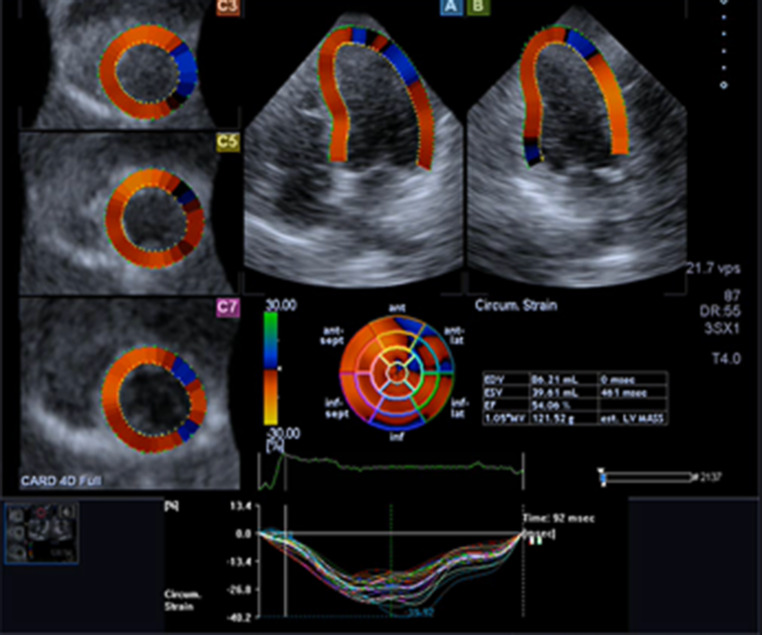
Measurements of the left ventricular (*LV*) volumes and function using three-dimensional speckle tracking echocardiography. Example of multiple long- and short-axis views extracted from a three-dimensional echocardiography data set at end-systole in a patient with normal systolic LV function. Visual information on circumferential (*Circum.*) strain is shown in colour overlay superimposed on grey-scale images (*EDV* end-diastolic volume, *ESV* end-systolic volume, *EF* ejection fraction)
